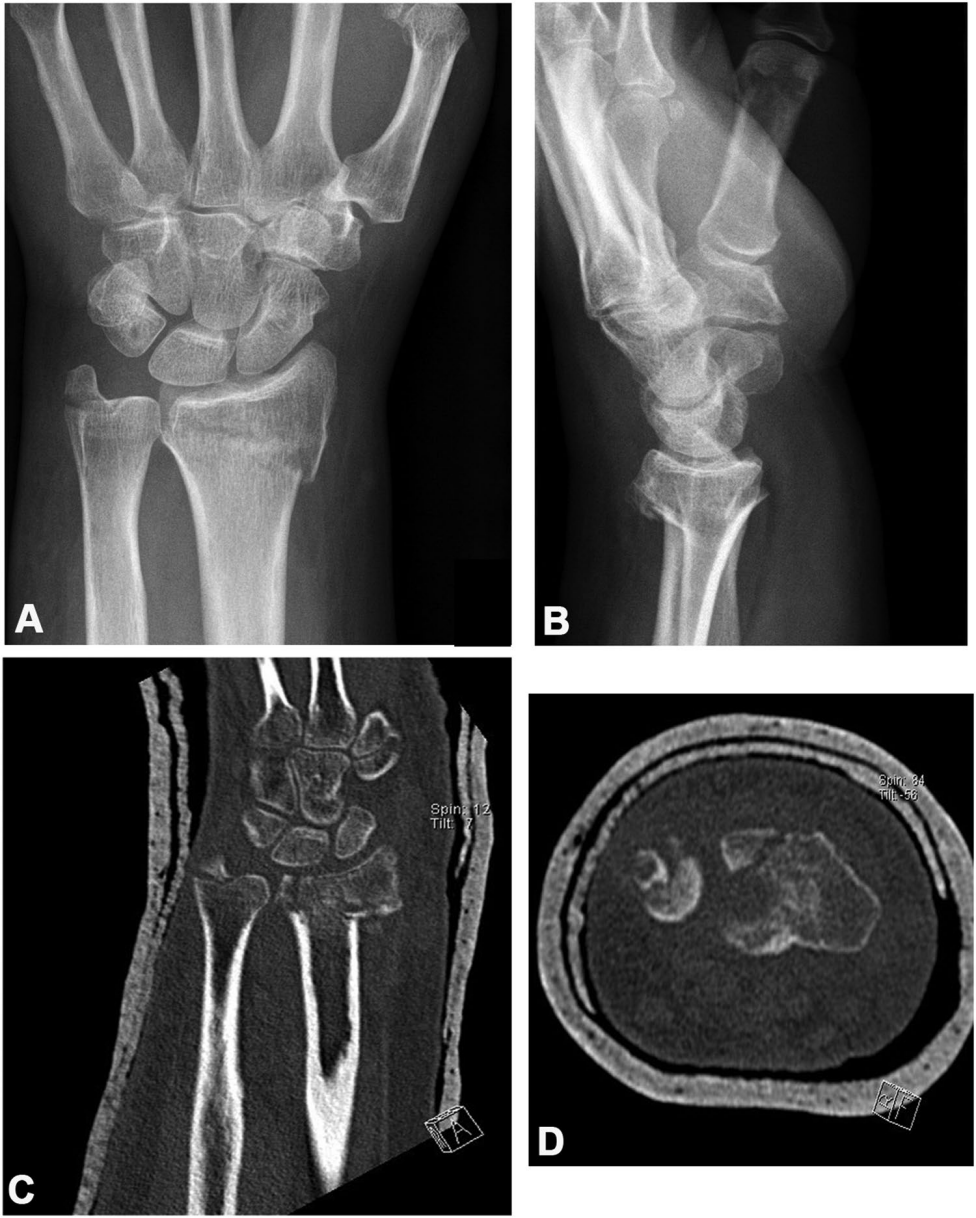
Distal radius fracture in a 63-year-old female patient. Considering the posteroanterior (a) and lateral (b) projections of plain film radiography, this fracture was classified as an extraarticular A2 fracture according to the AO/OTA classification. In the coronal (c) and axial (d) CT reconstructions, however, radiocarpal joint involvement was clearly identified
